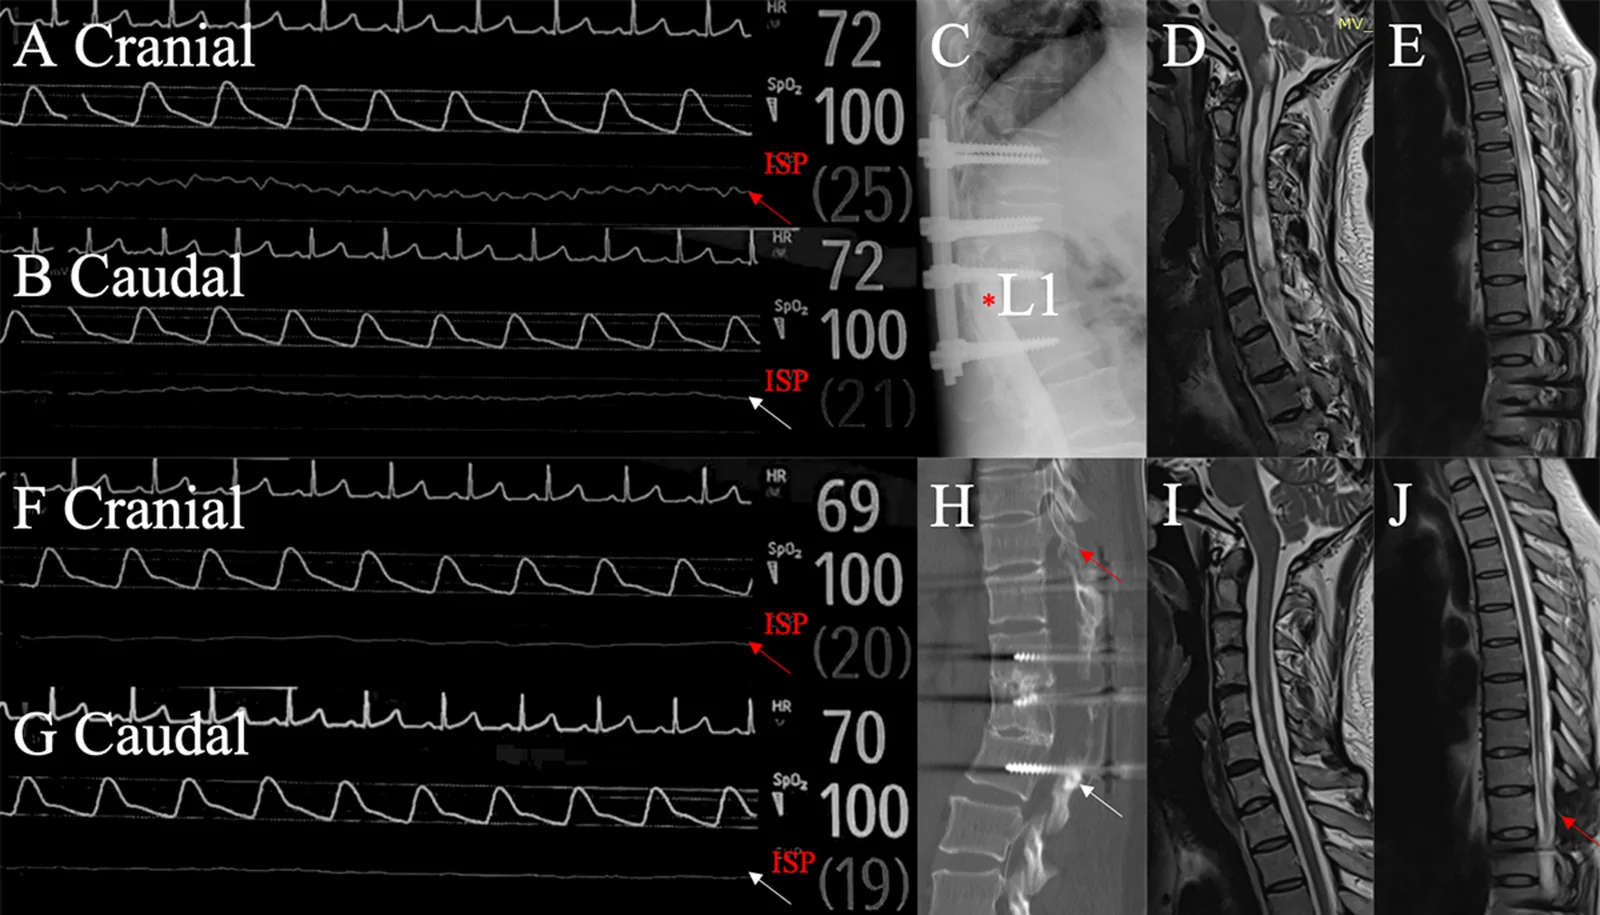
A 30-year-old male presented with pain and numbness in the left upper extremity for two years. His history included a SCI at the T12–L1 level sustained in a traffic accident fifteen years prior. CASE2: (A, B) Preoperative intraspinal pressure (ISP) tracings cranial (A, red arrow) and caudal (B, white arrow) to the arachnoid adhesion at L1 demonstrate elevated pressure gradient with abnormal waveform morphology. (C) Lumbar myelogram confirming a complete subarachnoid block at the L1 level (red asterisk) due to dorsal arachnoid scarring. (D, E) Preoperative T2-weighted magnetic resonance (MR) images reveal a large syrinx extending rostrally to the medulla oblongata. (F, G) Postoperative ISP tracings at corresponding locations (F: cranial, G: caudal) after S-S bypass placement show stable waveforms without a significant change in the pressure gradient. (H) Postoperative computed tomography (CT) localizer image confirming the position of the S-S bypass conduit spanning from T11 (red arrow) to L2 (white arrow). (I, J) Postoperative T2-weighted MR images demonstrate marked regression of the syrinx
